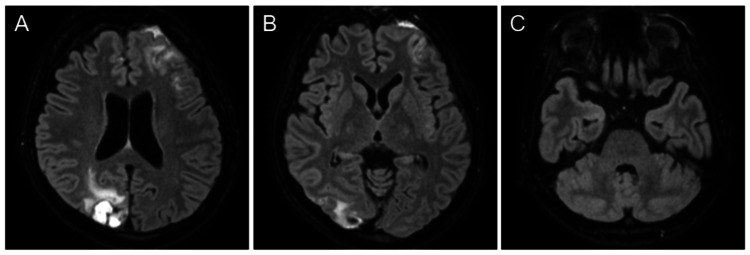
Brain magnetic resonance imaging on the 29th day of hospitalization. (A-C) Fat-suppressed three-dimensional fluid attenuated inversion recovery images reveal that the lesions exhibit a tendency to resolve.
